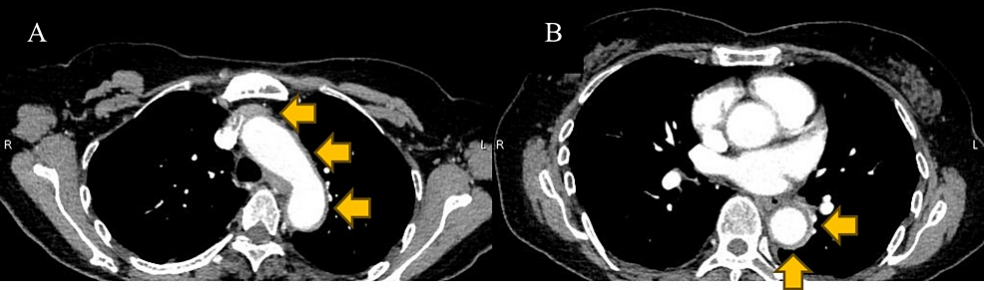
Contrast-enhanced CT scan showing wall thickening of the aortic arch (A) and descending aorta (B) on day 17.
